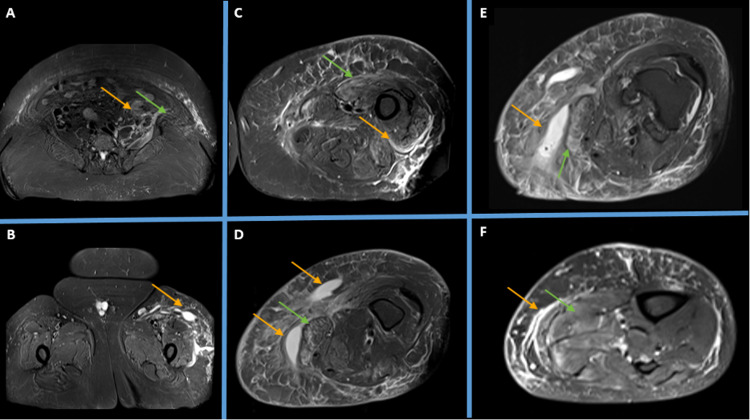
Day 36 MRI Axial short tau inversion recovery (STIR) images at the level of (A) pelvis, (B) proximal left thigh, (C) mid left thigh, (D) lower left thigh, (E) left knee joint, and (F) proximal left leg, with orange arrows showing persistent infective fluid pockets and green arrows showing interval infective myositis.
